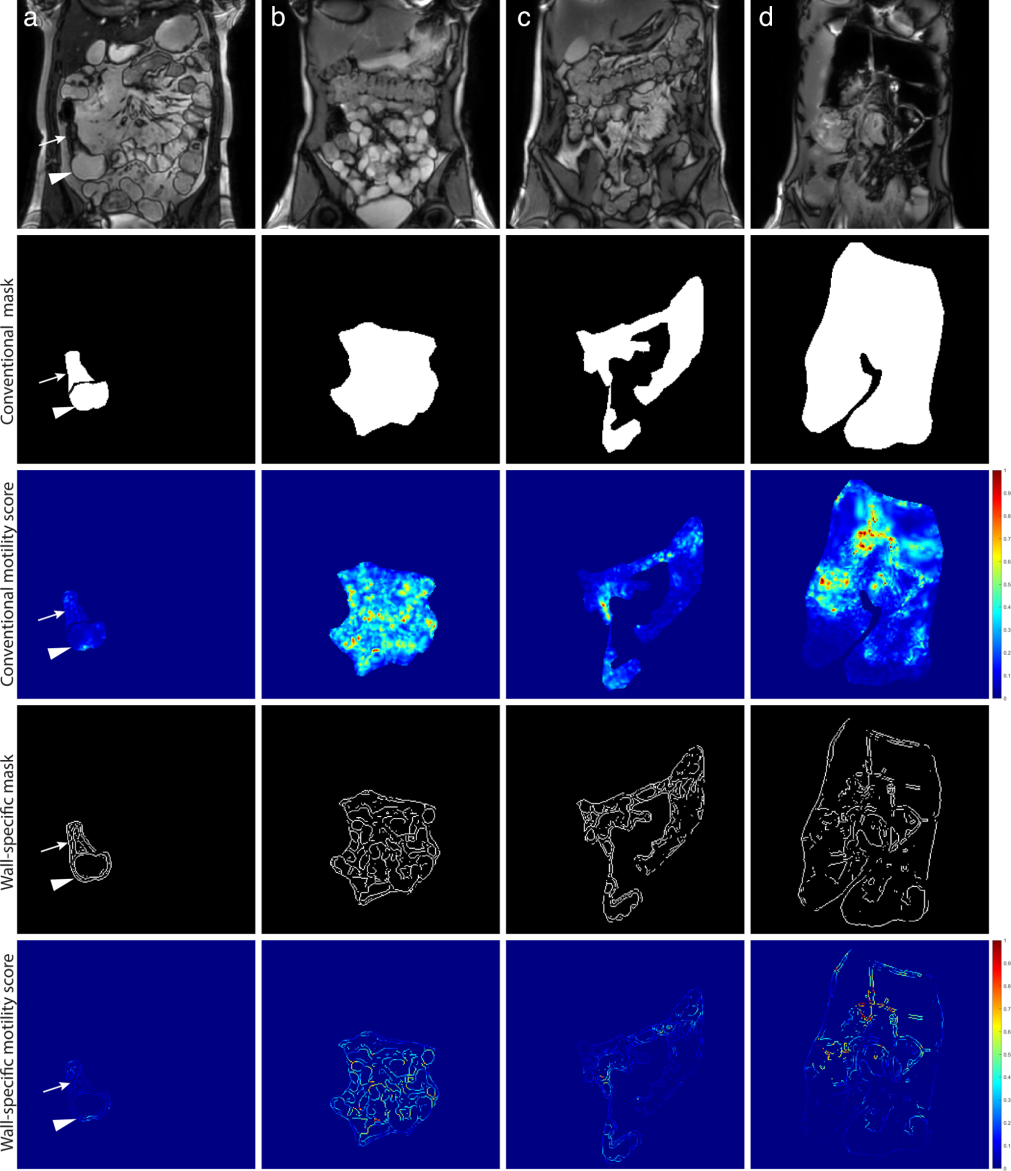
MRI examples, binary masks and motility maps of the four included populations. The first row shows the reference MRI scans of the different populations, (a) shows a Crohn’s disease patient with stricture (tailed arrow) and prestricture dilation (arrowhead), (b) a mannitol-prepared healthy subject, (c) a fasted healthy subject and d) a CIPO patient with dilated bowel loops. The second row shows the conventional binary masks and the third row the corresponding motility maps from which the conventional motility score was calculated. The fourth row shows the wall-specific binary masks and the fifth row show the motility maps from which the wall-specific motility scores were calculated. For the Crohn’s patients, a motility score for the stricture and motility score for the prestricture dilation were calculated. Areas of high motility are shown in red and low motility in blue.
